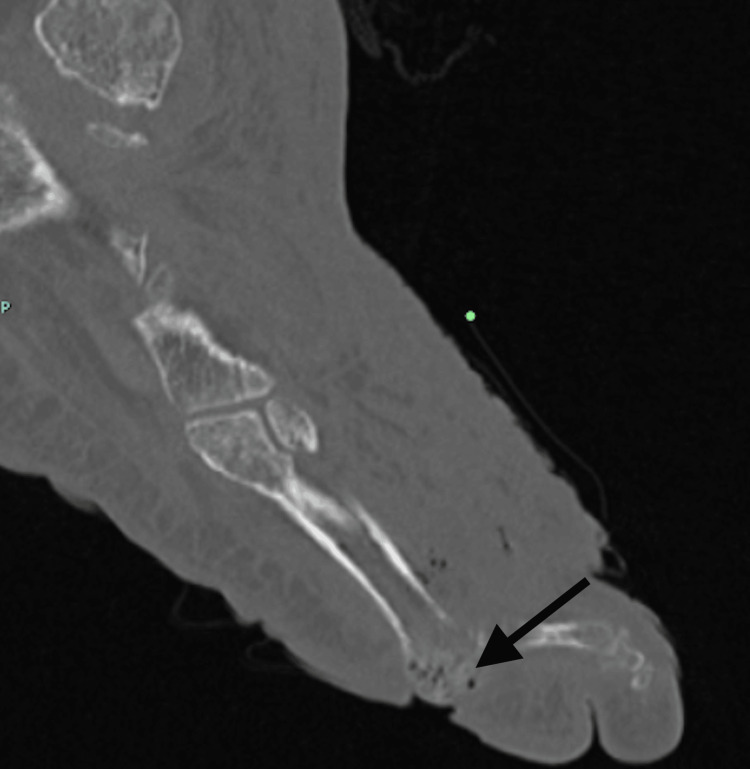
Emphysematous osteomyelitis of the left fifth metatarsal head. A CT of the left foot axial view in a 57-year-old man presenting with left lower extremity pain showing emphysematous osteomyelitis (black arrow).
